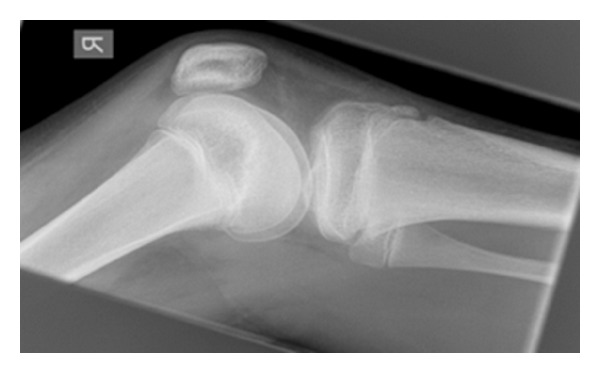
Lateral radiograph of the right knee taken seven weeks after injury.
